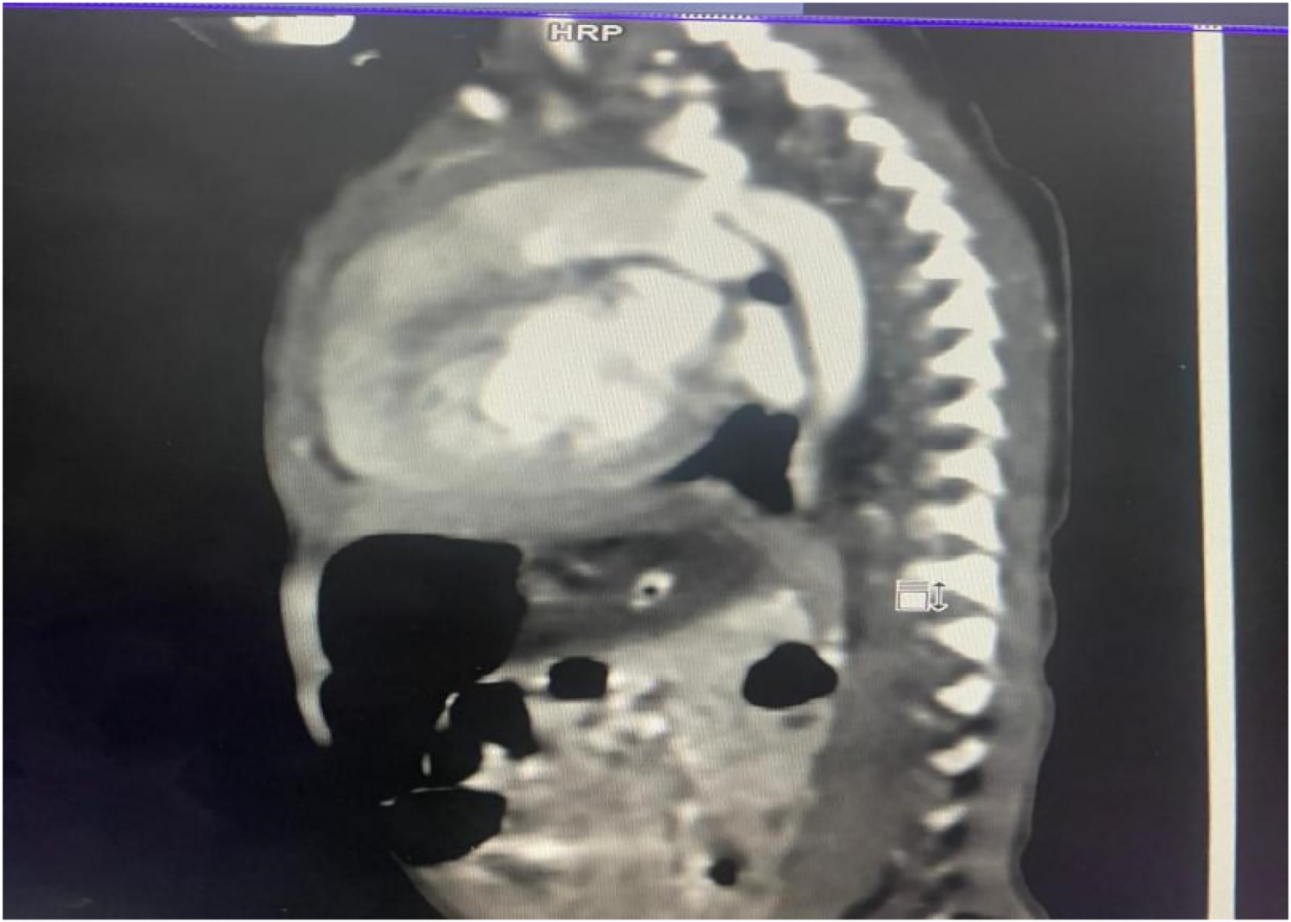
CT aortogram sagittal view after ballooning.
